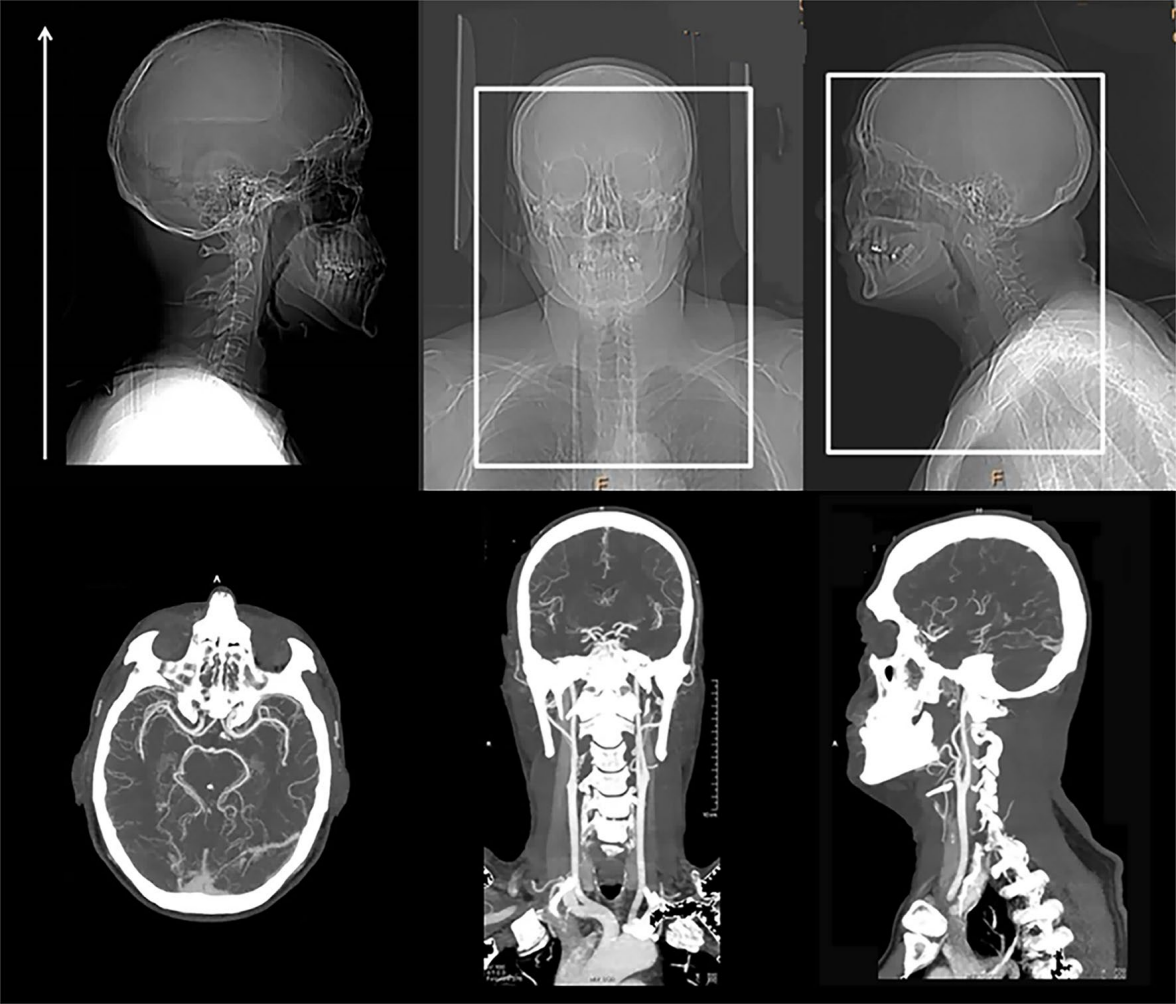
Single-phase CT angiography covers from the aortic arch to vertex and maximum intensity projection reconstructions for the visualization of cervical and intracranial vessels. CTA of the cervical and intracranial vessels was performed as follows: 0.7 mL/kg contrast (maximum 90 mL), 5- to 10-s delay from injection to scanning, 120 kV, 270 mA, 1 s/rotation, 1.25-mm-thick slices, and table speed 3.75 mm/rotation. The axial images were reconstructed at 1-mm overlapping sections, and multiplanar reconstructions for axial, coronal, and sagittal images of the circle of Willis were performed with 3 mm thickness at 1-mm intervals. Thick-section axial maximum intensity projections at 24 mm thickness and 4-mm intervals were also reconstructed
